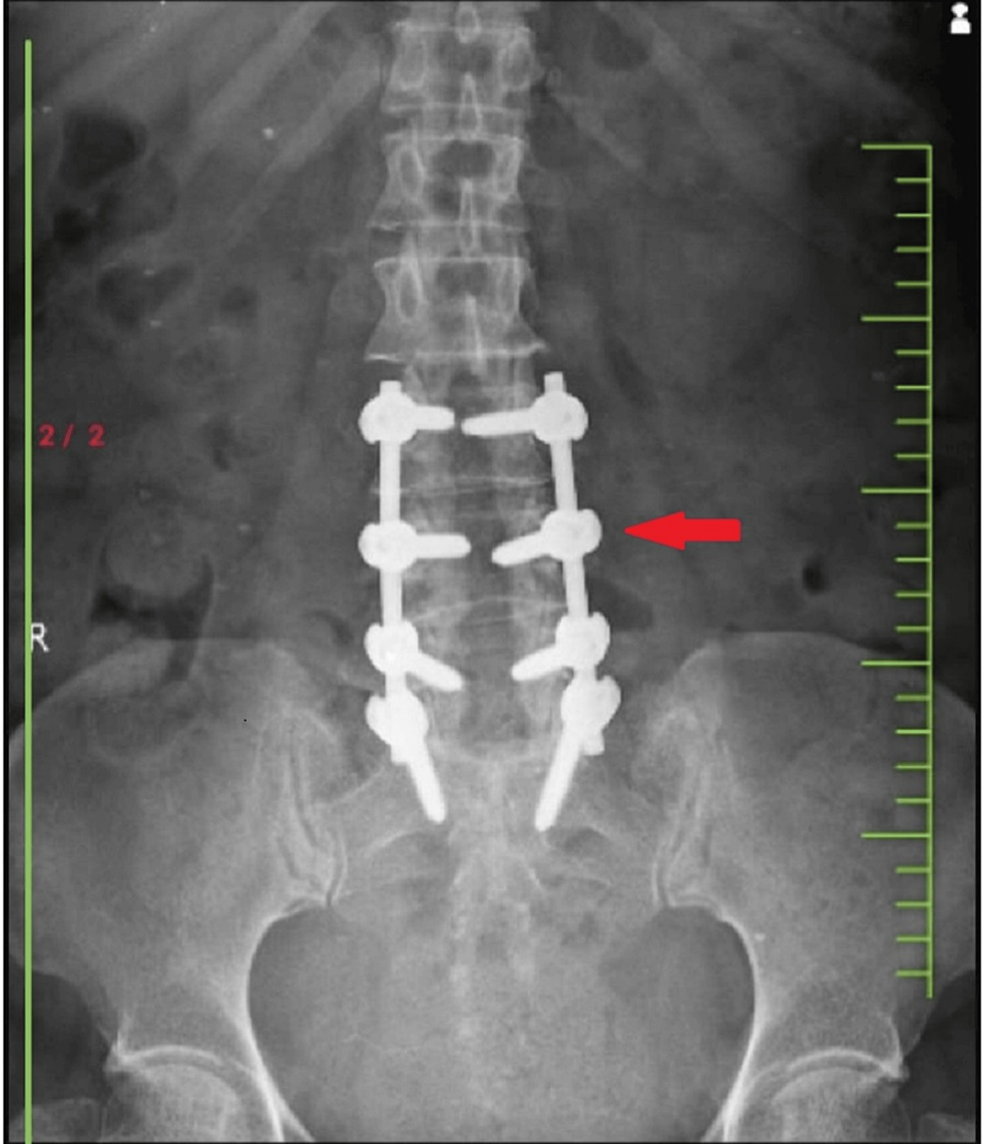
Post-operative CT scan of the lumbar spine after lumbar decompression and spinal fusion at level L3 to S1 using Pedicel screw (red arrow) CT - computed tomography
